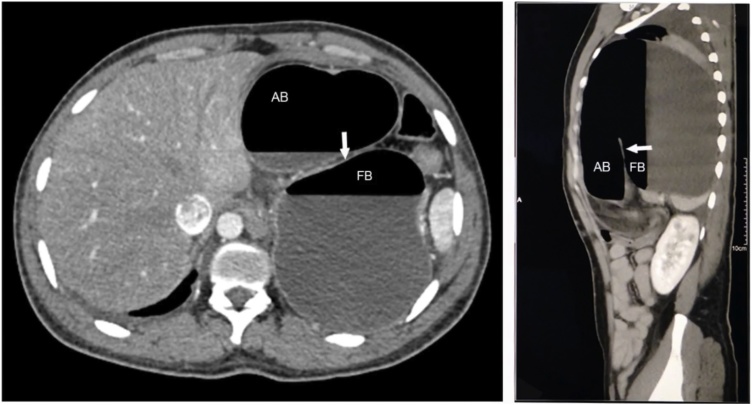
CT showing the gastric volvulus.
